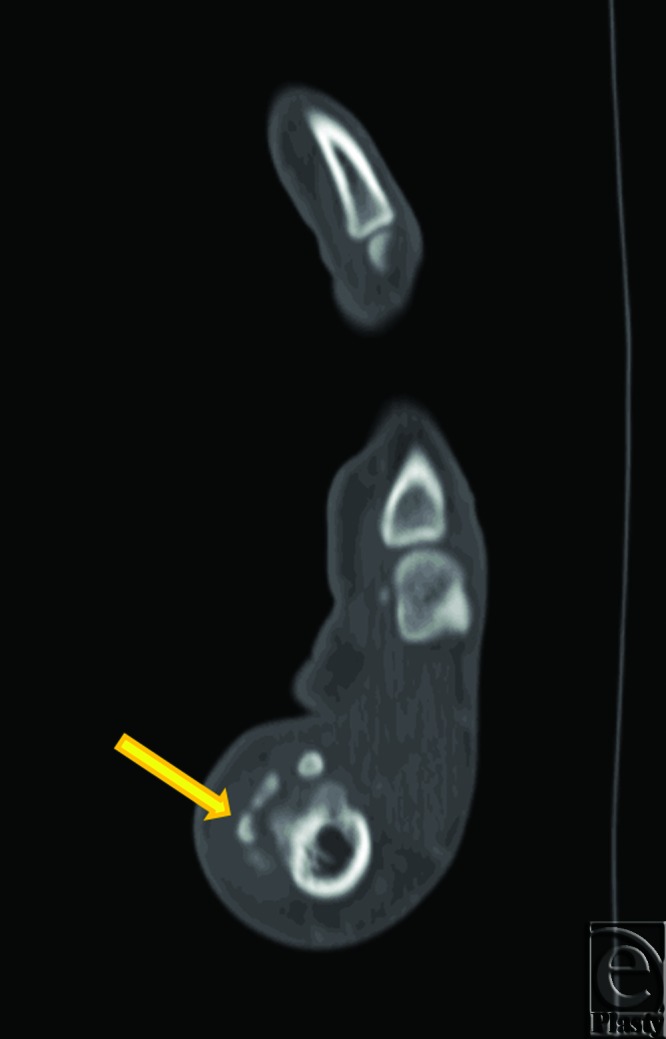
Computed tomography. Foci of mineralization (arrow) are again seen located adjacent to the volar and radial aspect of the right first metacarpophalangeal joint. Based on the x-ray and CT images, a radiographic diagnosis of synovial chondromatosis was suggested.
